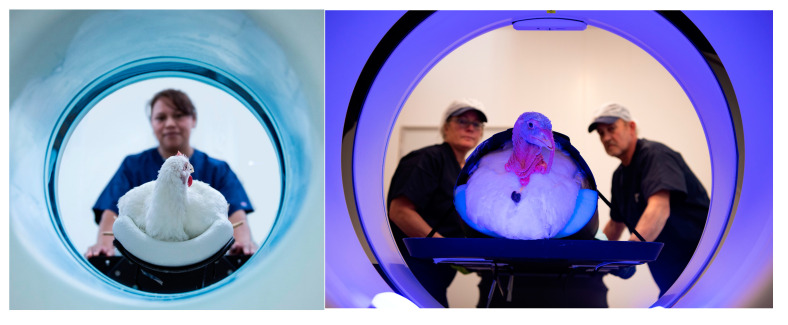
CT scanning of broilers (Left) and turkeys (Right).
